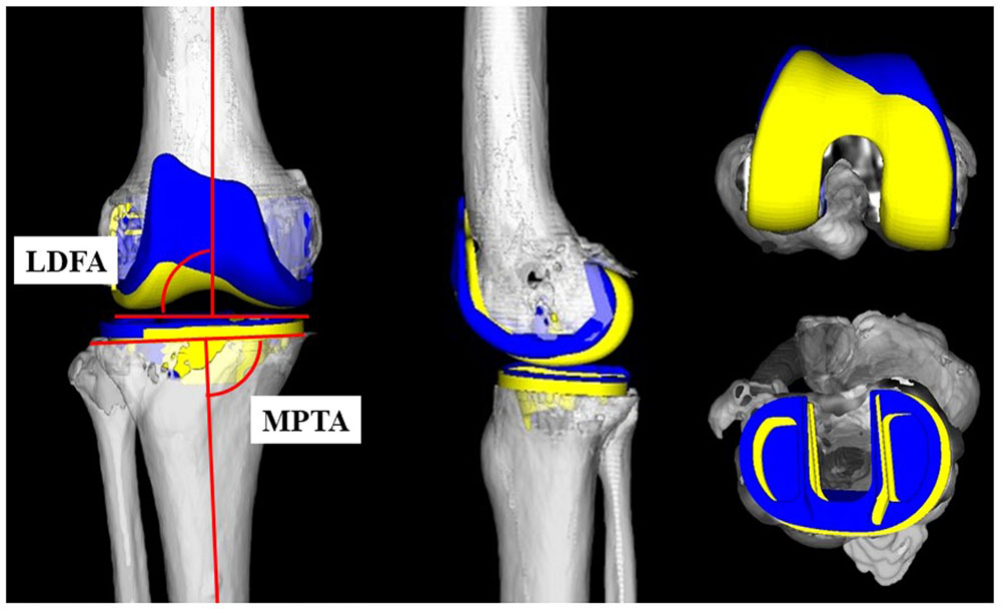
Pre‐operative plan three‐dimensional computed tomography (3DCT) plan and post‐operative 3DCT images were shown. Three‐dimensional computer‐aided design data of femoral and tibial components were fit to the 3DCT plan and images. Blue components (preoperative plan image), Yellow components (post‐operative component). LDFA, lateral distal femoral angle; MPTA, medial proximal tibial angle.
